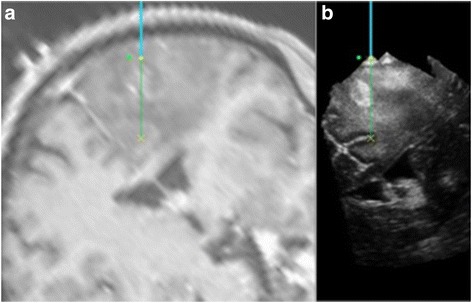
An example of an oligodendroglioma with a transformed region. The transformed region is demonstrated by the enhancement on the navigation T1 with contrast MRI (movement artefact of the patient) (a) and the corresponding hyperechogenic area on the i3DUS image (b). On these images, the navigation tool is represented by the *blue line* and its tip by the *yellow circle*. The projected tip extension is shown as a continuation of this line
